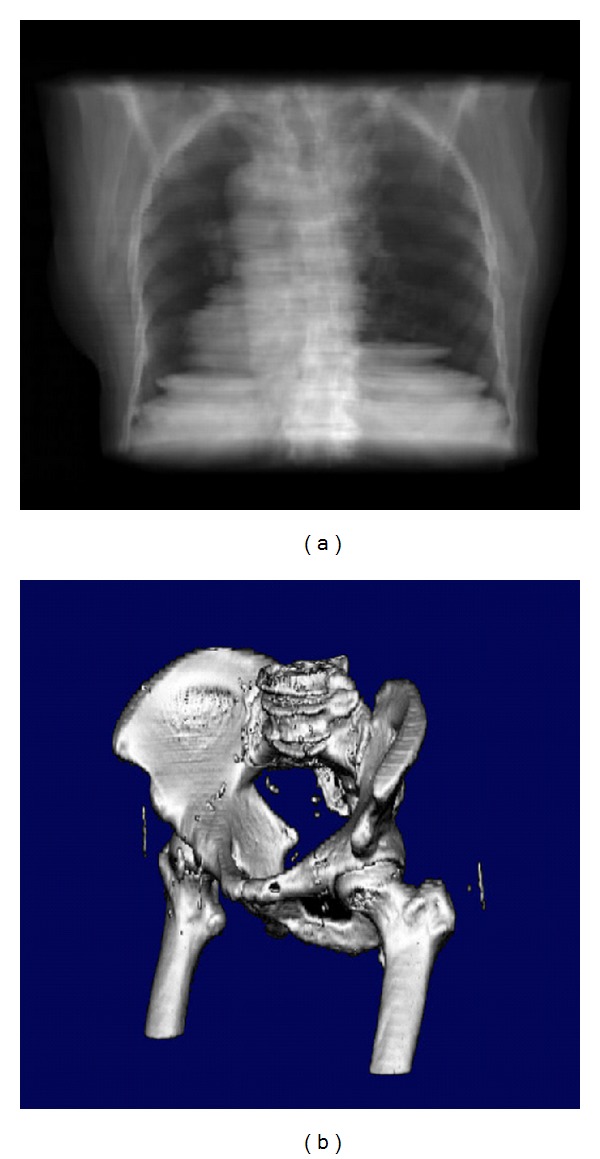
(a) Reconstruction of CT volume using DRR; (b) Reconstruction using direct volume surface using direct gradient volume estimation [21, 28, 33].
